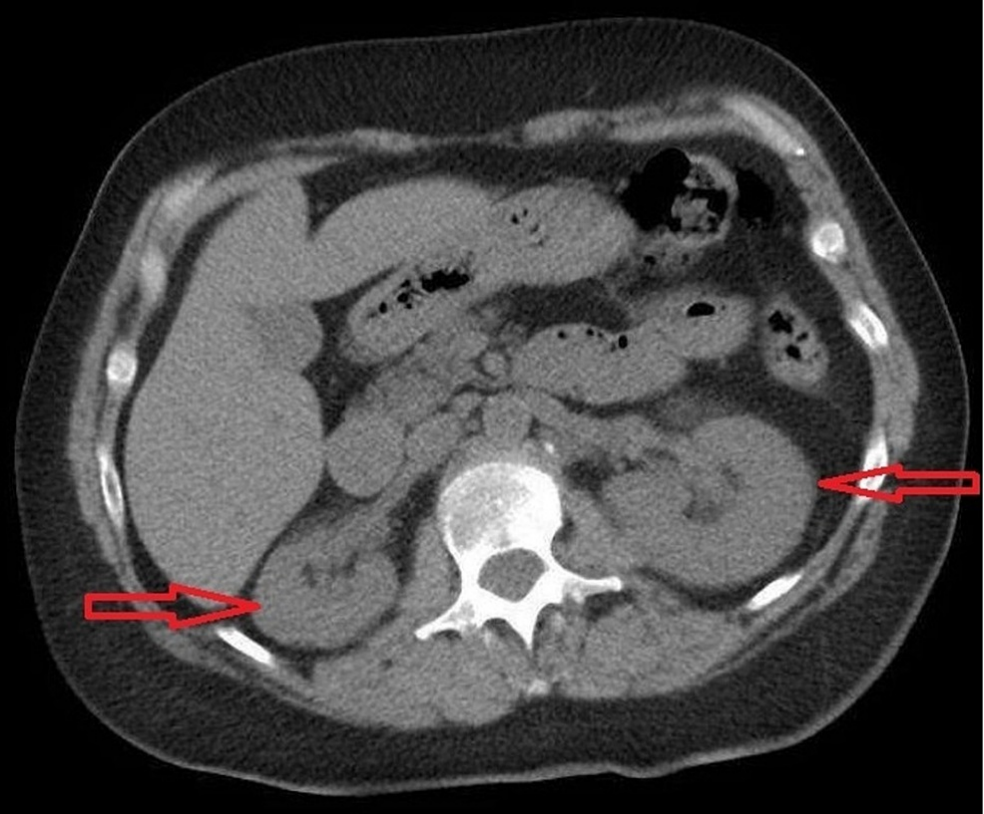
Computerized tomography (CT) abdomen and pelvis without contrast revealed no urolithiasis or hydronephrosis
